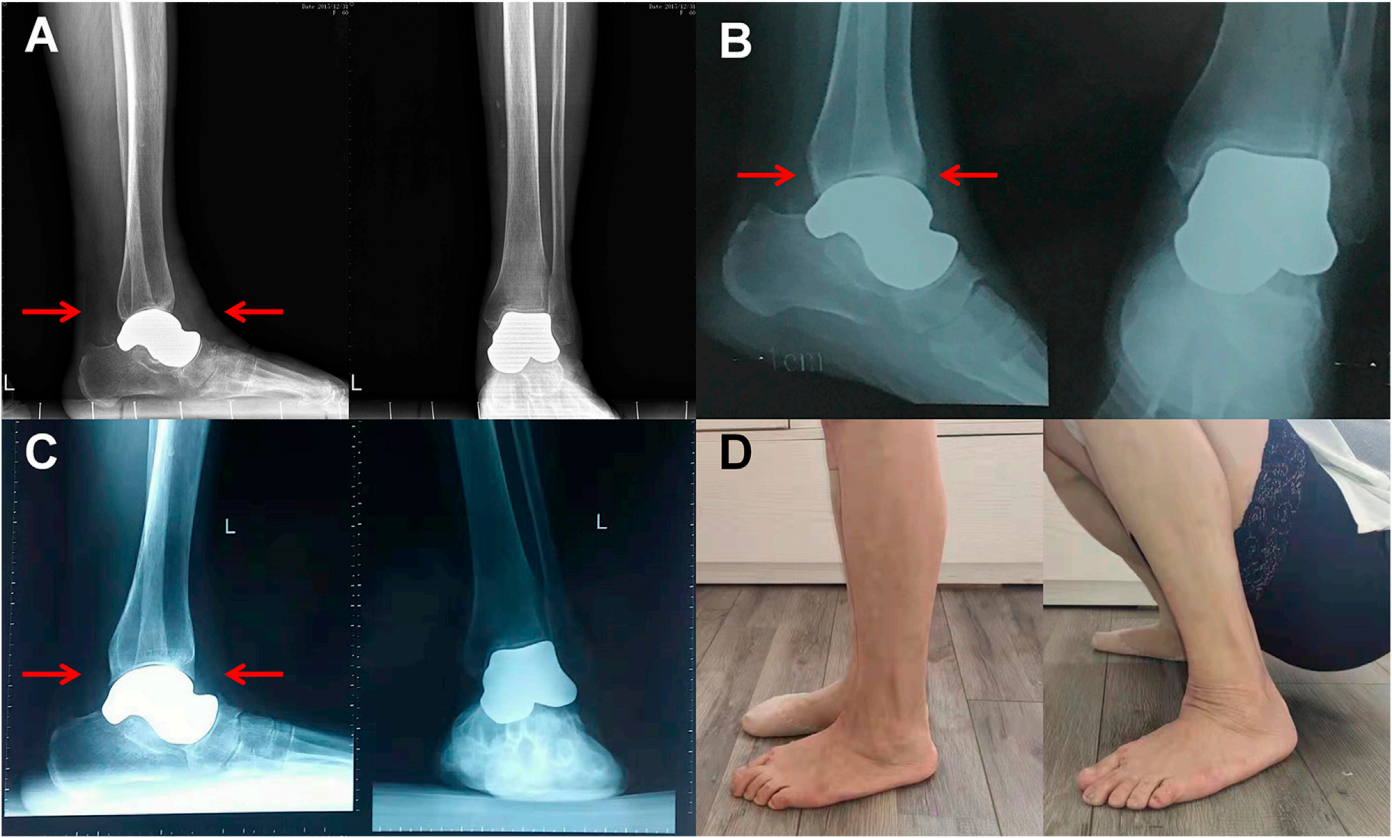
A 58 year-old woman (patient 1) suffered from pain and swelling of the left ankle for 2 years and was unable to walk without crutches. TTR with a 3D printed Vitallium talar prosthesis was performed in 2015. Anteroposterior and lateral radiographs taken postoperatively 1, 3, and 5 years after discharge did not depict any signs of prosthesis-related complications (A–C). The formation of small osteophytes on the tibial side and navicular side was detected (D), but the patient did not report any discomfort and had returned to daily activities.
